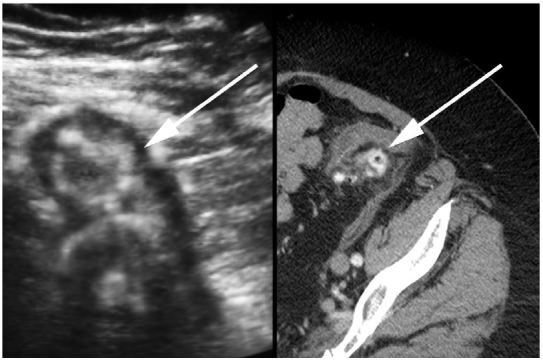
Transabdominal sonographic view of the patient’s abdomen (Left) and associated axial computed tomography (Right) revealing inflamed diverticula (arrow). Related imaging findings of diverticulitis such as edematous diverticula with thickened hypoechoic walls and hyperechoic centers, and surrounding hyperechoic zones representing inflamed fat.
